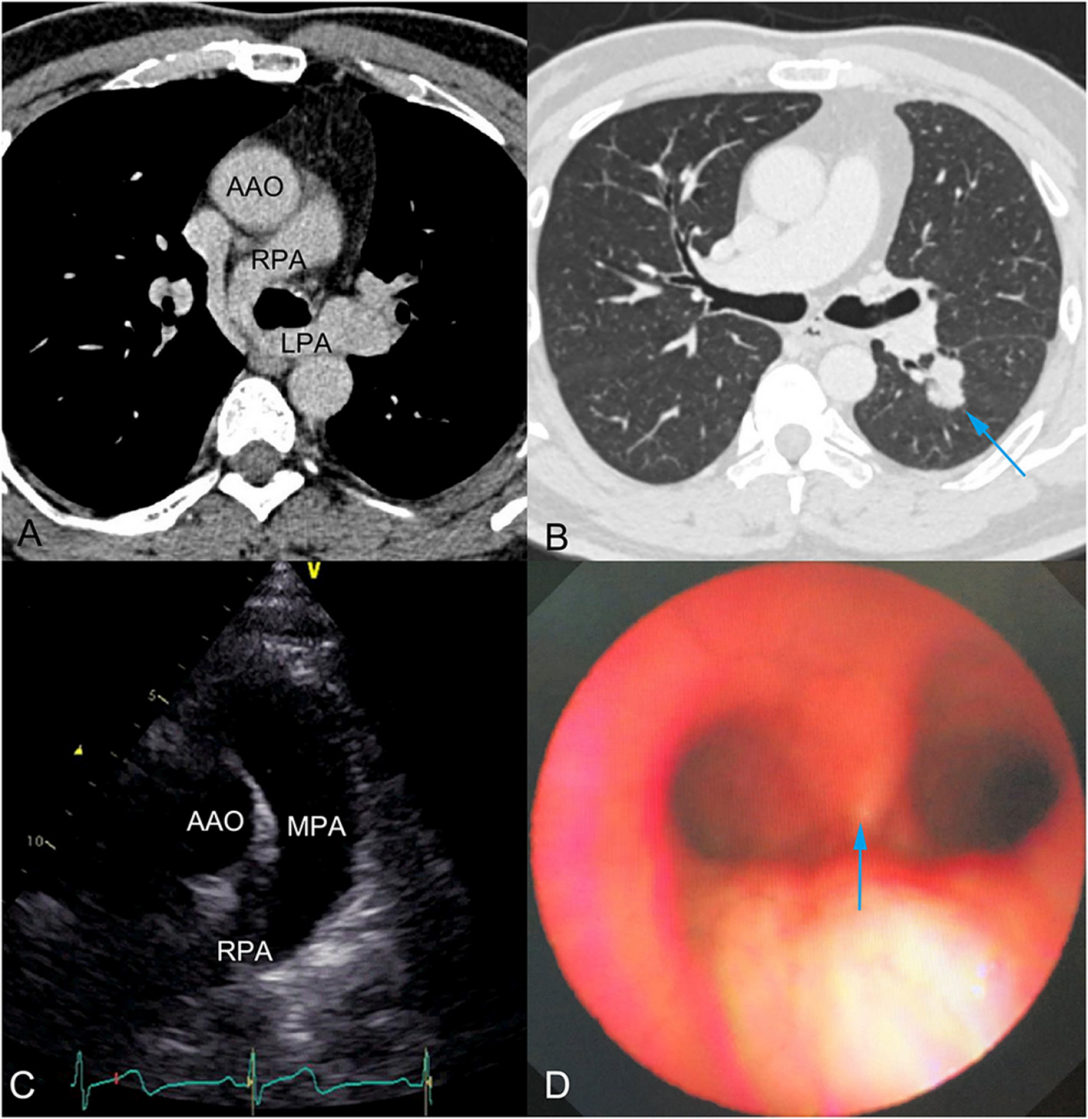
(A) Chest computed tomography (CT) showed pulmonary artery sling, which was observed for the first time during his life. (B) Preoperative chest CT showed a nodule of 2.5 cm diameter and indicated the possibility of malignancy (blue arrow). (C) Transthoracic echocardiography showed the main pulmonary artery had only one outflow tract to the right pulmonary artery. The beginning of the left pulmonary artery could not be detected, due to its deep position. (D) Bronchoscopy showed no obvious tracheal stenosis or bronchomalacia. Blue arrow indicates the tracheal carina. AAO, Ascending aorta; RPA, Right pulmonary artery; LPA, Left pulmonary artery; MPA, Main pulmonary artery.
